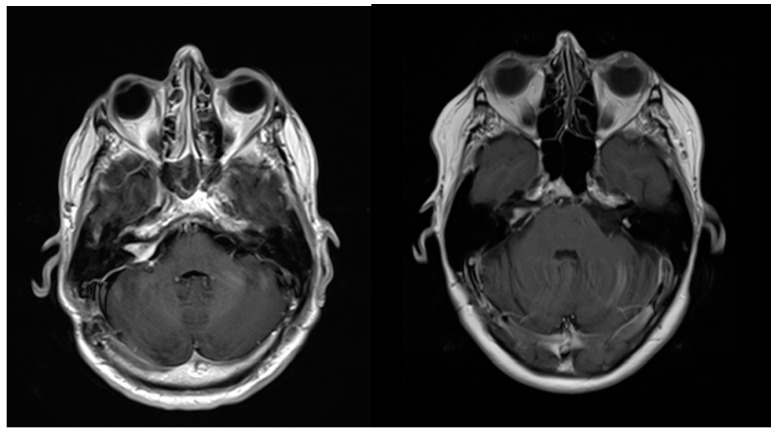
Patient No. 1. Enhanced magnetic resonance image showing right-sided vestibular schwannoma 6 months after planned subtotal resection. No increase in tumor volume was observed at 57 months of follow-up (right-sided image).
